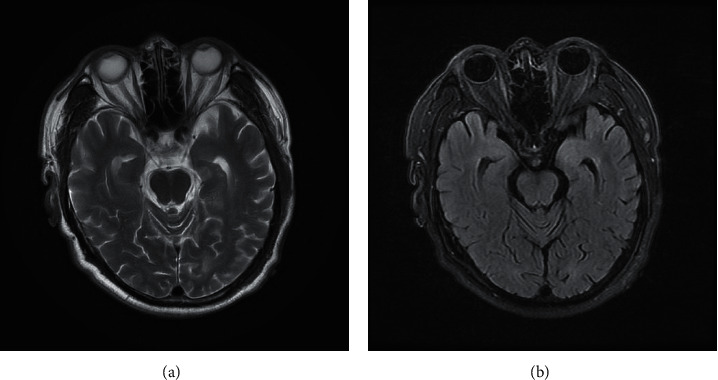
The cranial brain MRI of one case of anti-GABAB receptor encephalitis showed bilateral hippocampal swelling.
